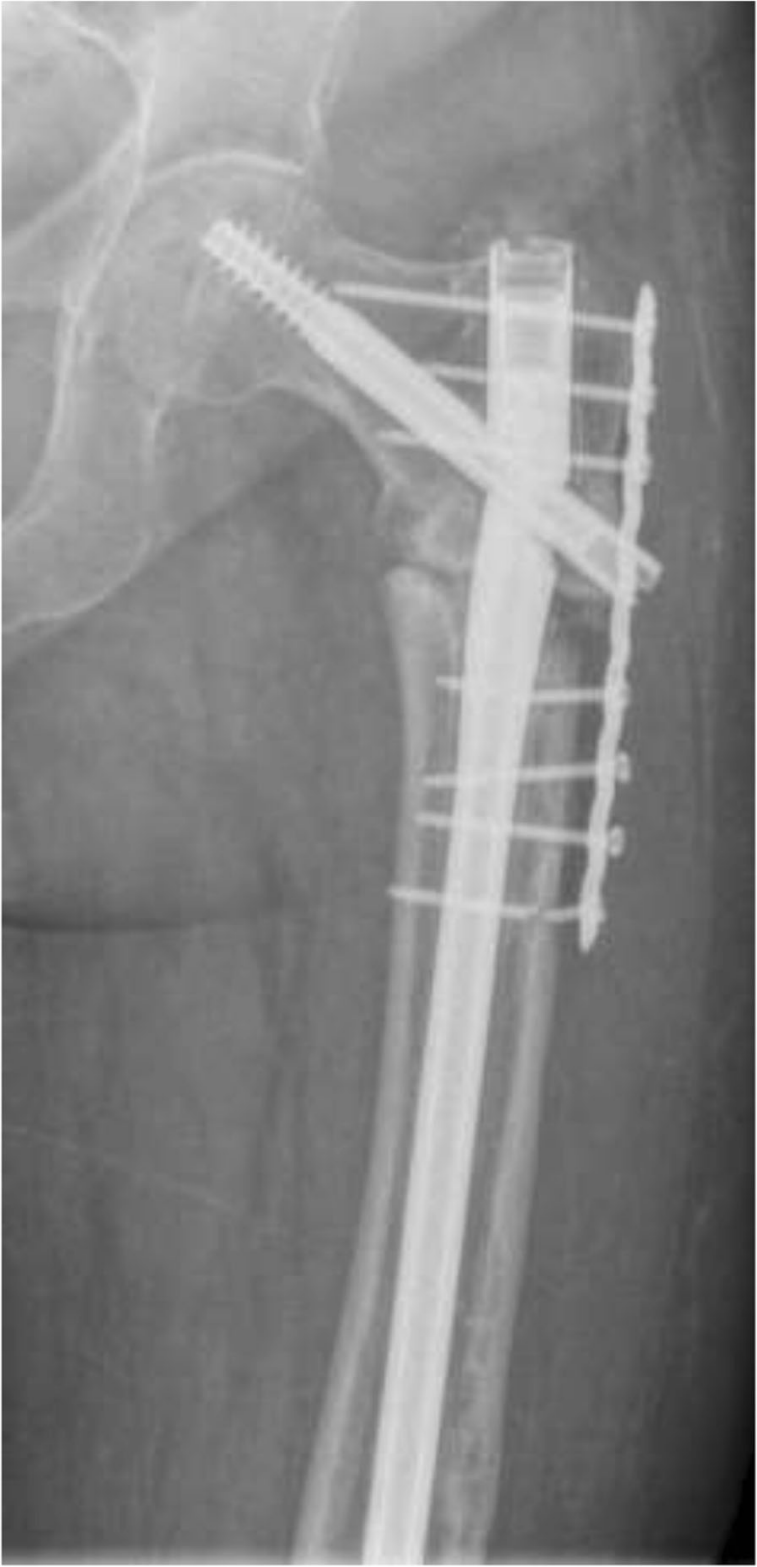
An 86-year-old female patient with a persistent femoral nonunion after nailing and having already undergone revision with augmentation plating. There is a lack of callus formation and radiographic breakage of the nail and two screws with varus deformation of the femur with a CCD of 104
